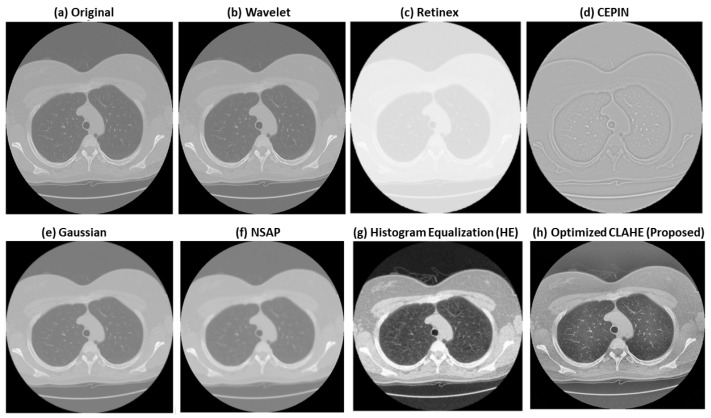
Visual comparison of the investigated image enhancement techniques on a representative CT slice: (a) Original, (b) Wavelet, (c) Retinex, (d) CEPIN, (e) Gaussian, (f) NSAP, (g) Histogram Equalization (HE), and (h) Optimized CLAHE (Proposed). The compared methods show different trade-offs between contrast enhancement, noise suppression, and structural preservation. The proposed optimized CLAHE provides the most balanced visual improvement, with clearer local contrast and better preservation of anatomical detail.
